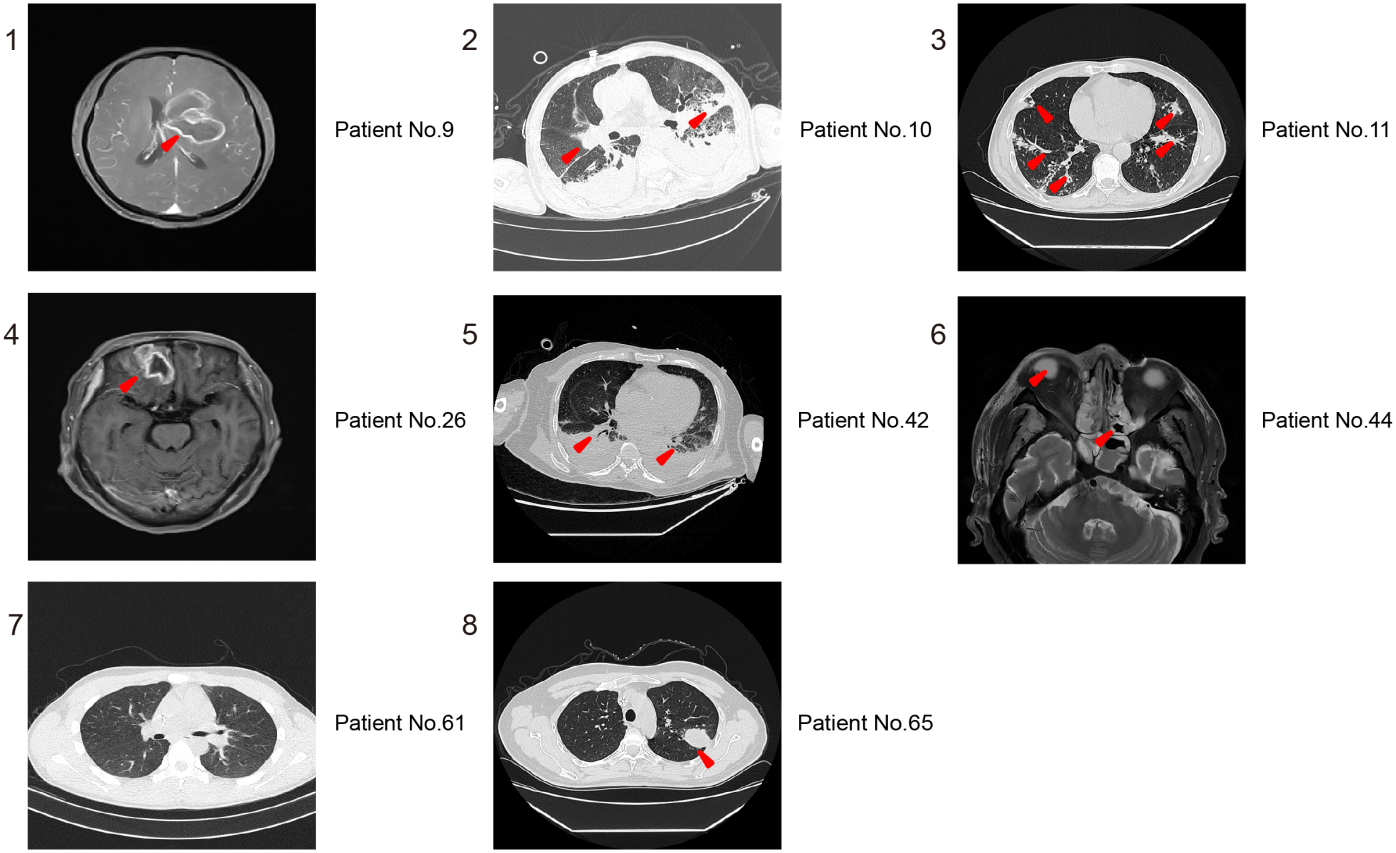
Representative imaging results for distinguishing *Mucorales* infection from colonization. 1,4, and 6 are MRI of Brain; 2, 3, 5, 7, and 8 are CT of lung. The red arrowheads showed the abnormal lesions.
